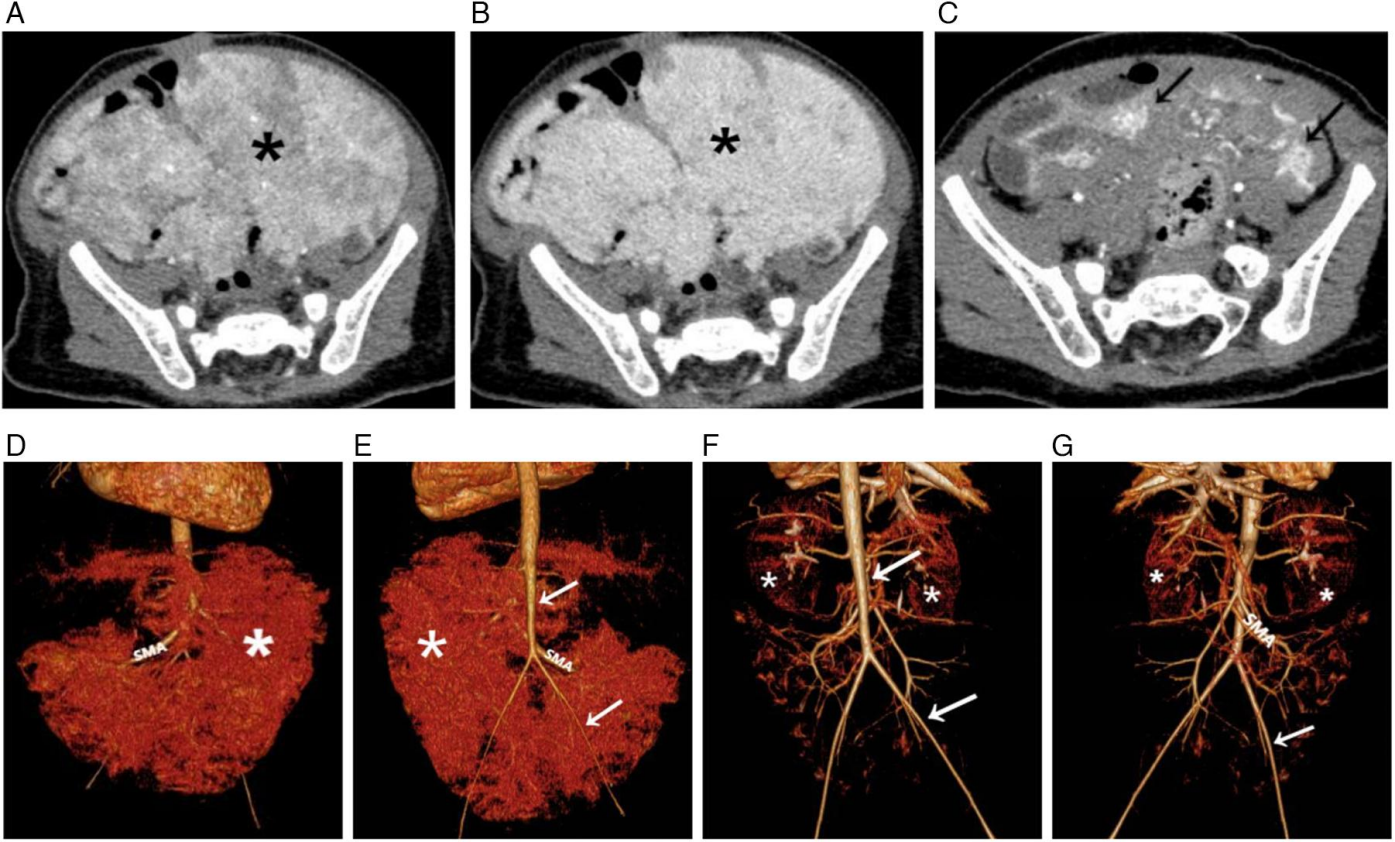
Contrast-enhanced CT, before and after treatment. (A,B) Pre-treatment axial CT images show a massive infiltrative mass (*) encasing mesenteric vessels and bowel loops, exhibiting heterogeneous arterial phase enhancement (A) and persistent, near-homogeneous venous phase enhancement (B,C) Post-treatment axial CT image reveals marked regression of the mass with only residual patchy enhancement (→). (D,E) Pre-treatment volume-rendered (VR) CT images depict the large hypervascular mass (*), a markedly dilated superior mesenteric artery (SMA), and significant narrowing of the distal aorta (→). (F,G) Post-treatment VR images confirm mass regression (*) and mild improvement in the previously noted arterial narrowing (→).
